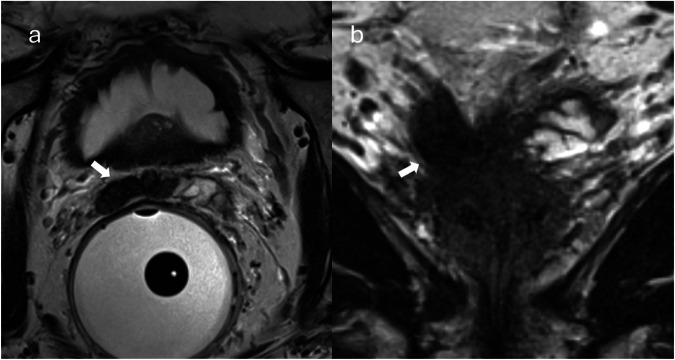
MRI-derived parameter for seminal vesicle invasion in MRI T-staging. Axial (a) and coronal (b) T2-weighted MR images demonstrating seminal vesicle invasion (SVI). MRI assessment of SVI relies on identifying direct tumour extension from the prostate base into the seminal vesicle (arrow), loss of the normal low-signal-intensity wall and replacement of the seminal vesicle lumen by intermediate to low tumour signal
